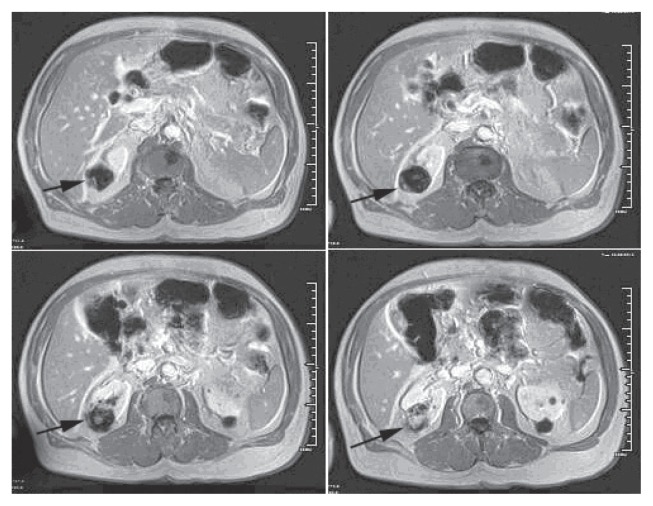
MR images with contrast showed a 4-cm complex enhancing mass at the projecting from the posterolateral aspect of the upper pole (indicating arrows) of the right kidney; the complex mass demonstrated heterogeneous signal internally including a focal hypointense signal on precontrastT1 weighted sequences; and these areas also showed hyperintense signal postcontrast suggesting hemorrhage, necrosis, and scar formation components.
